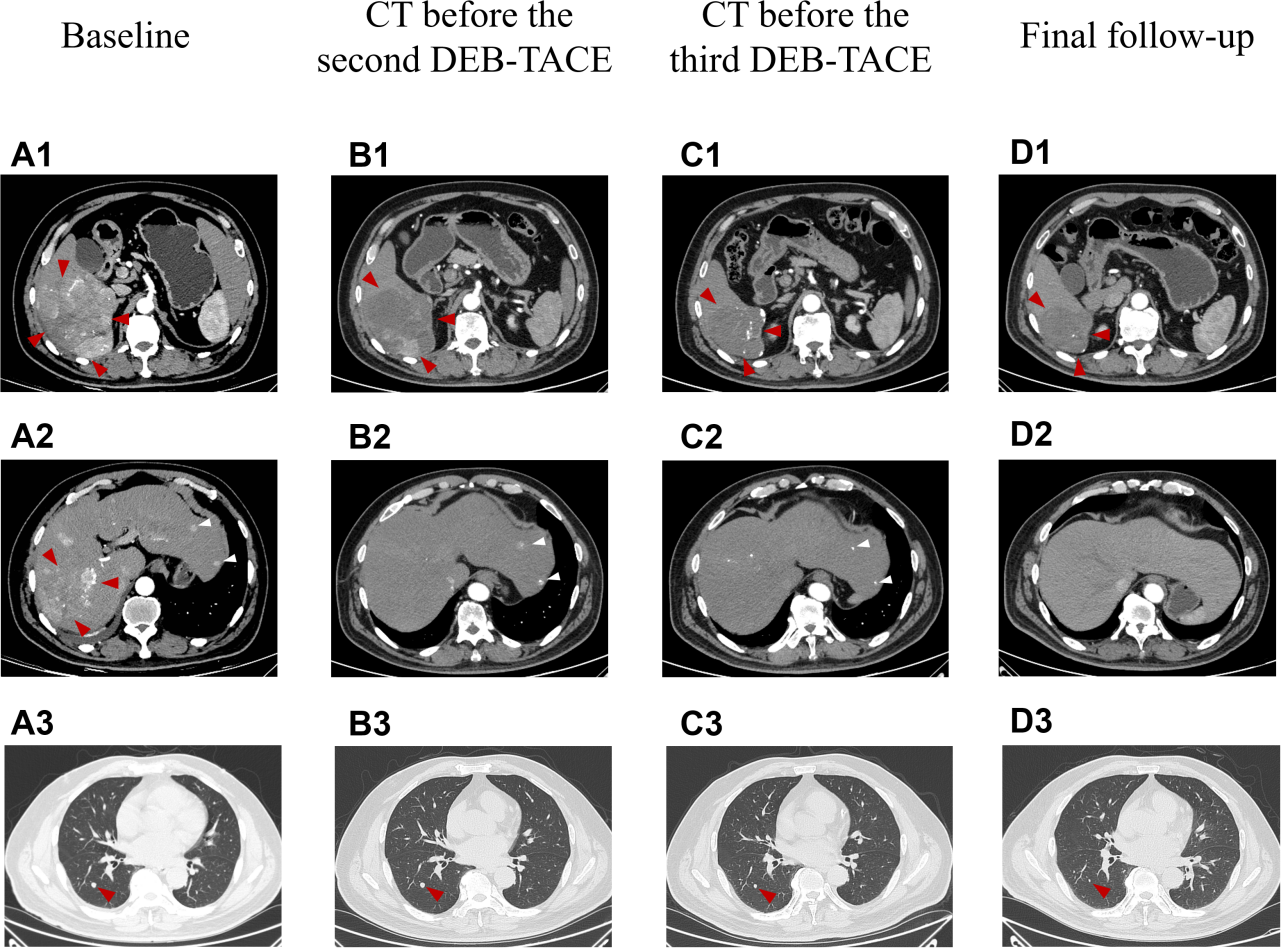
The representative chest and abdominal enhanced CT images changed during clinical treatment. (A1–A3) Before the first DEB-TACE treatment (at baseline), the abdomen enhanced CT showed a large lumpy blood-rich tumor in the right lobe of the liver, with a size of about 11.1 cm × 10.2 cm (shown by red arrows), multiple subfocies of HCC were also seen in the liver (shown by white arrows), and metastatic lesions in the dorsal segment of the lower lobe of the patient’s right lung (size about 0.7 cm × 0.7 cm); (B1, B2) Two months after the first DEB-TACE treatment (before the second TACE treatment), the massive tumor in the right lobe of the liver shrank (about 9.2 cm × 7.8 cm in size), and a large area of necrosis was visible in it, which was assessed as PR according to the mRECIST criteria (red arrows), and good iodized oil deposition was seen in the subfocies (white arrows); (C1, C2) Two months after the second DEB-TACE treatment (before the third TACE treatment), the original right lobe of the hepatic massive tumor (shown by red arrows) and intrahepatic subfocies (shown by white arrows) were inactive, and were assessed as CR according to mRECIST criteria; (D1, D2) Fourteen months after the first treatment (the final follow-up), the original hepatocellular carcinoma lesions in the liver were stable and inactive. (B3–D3) Two months after the first DEB-TACE treatment, two months after the second DEB-TACE treatment and 14 months after the first treatment.Chest CT images, the diameter of the dorsal nodule in the right lower lobe, 7mm, 6mm, 2mm, respectively. mRECIST, the Modified Response Evaluation Criteria in Solid Tumors.
